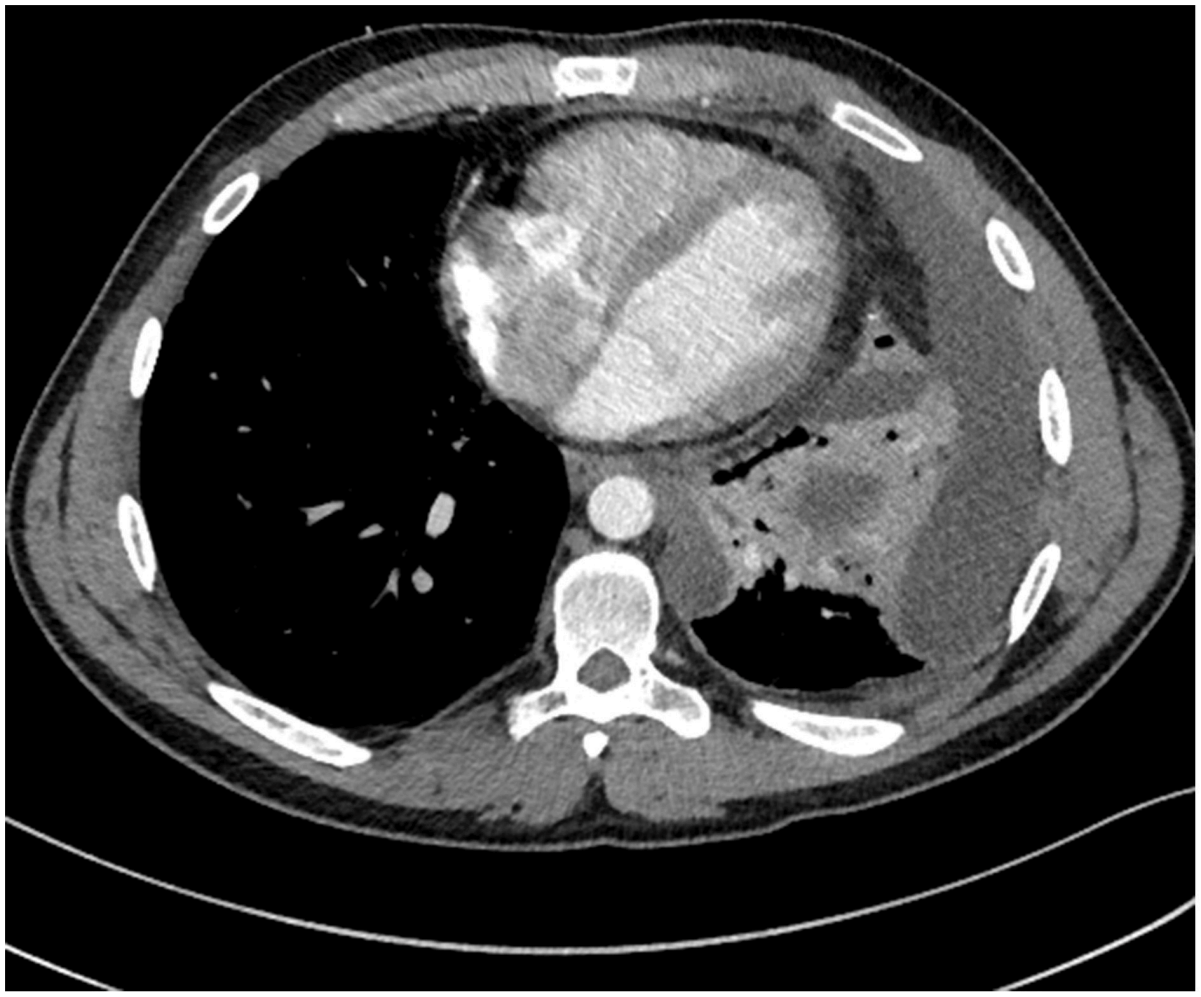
A computed tomography (CT) scan showed a multi-loculated left pleural effusion.
